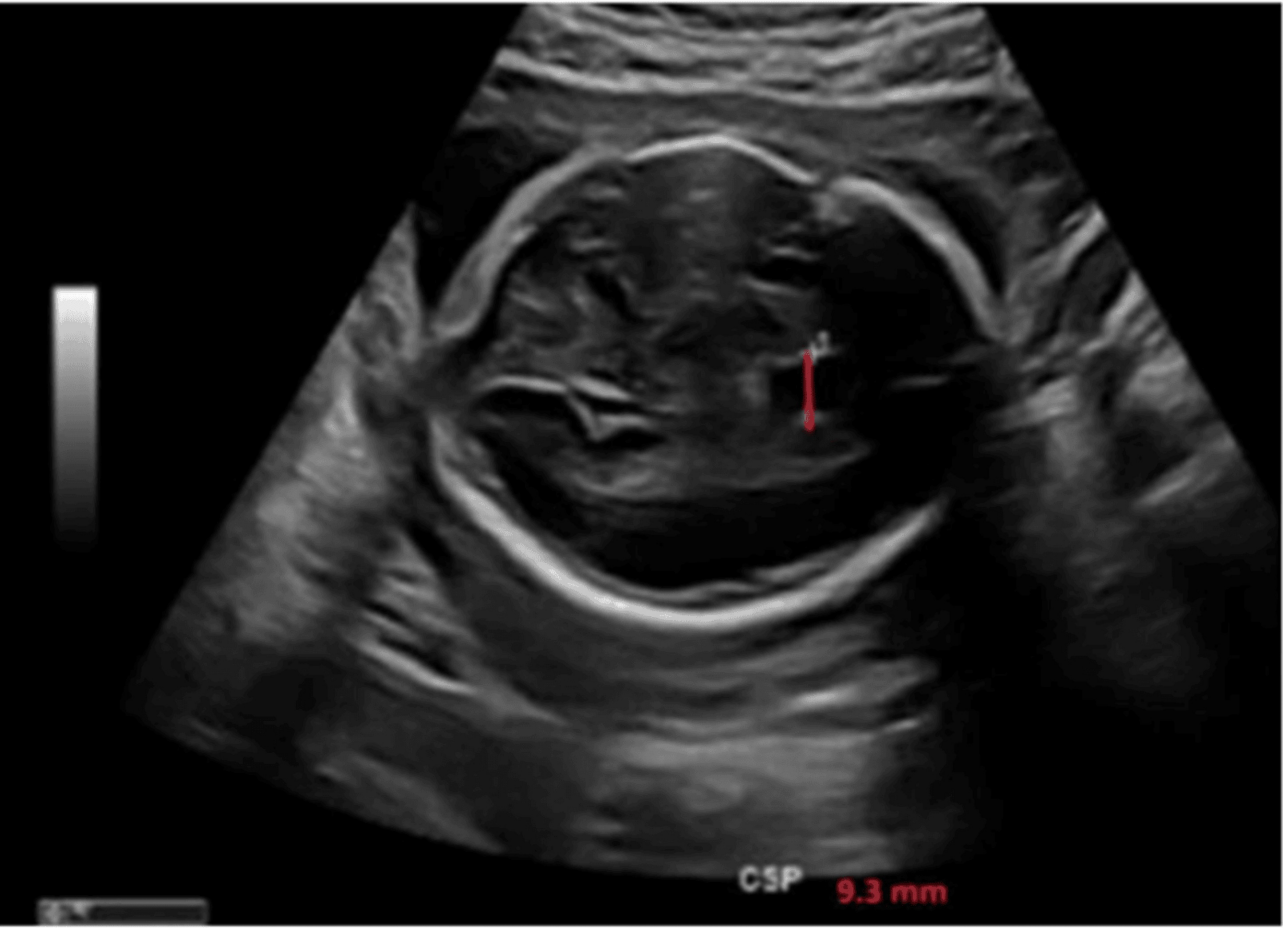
Repeat targeted ultrasound at 26 weeks and 4 days of gestation demonstrating interval enlargement of the CSP to 9.3 mm (red line). Other midline structures remain normal CSP: cavum septum pellucidum
